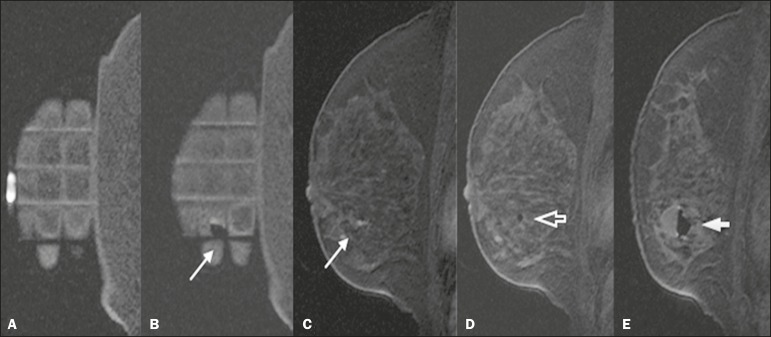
Sagittal fat-suppressed T1-weighted MRI scan of a 53-year-old woman
